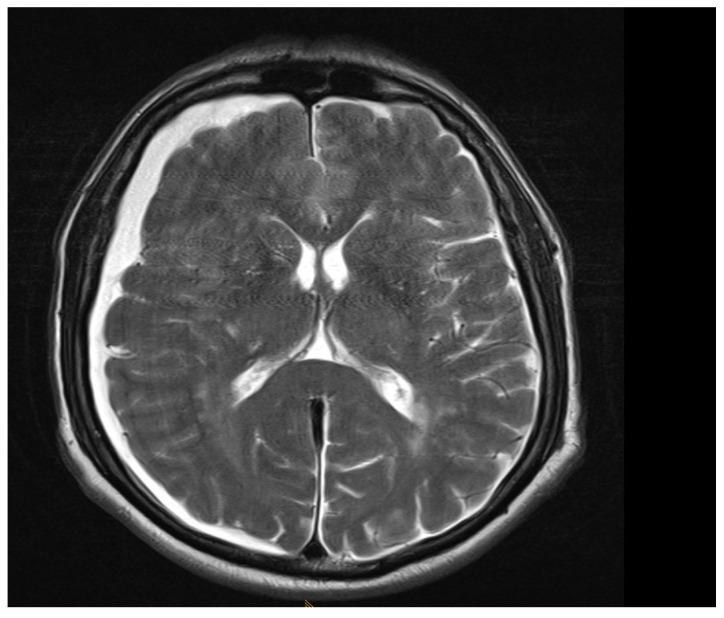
Axial T2 MRI, showing subdural hygroma on the right side. The image was acquired 1 month after intrathecal injection due to persistent headache.
